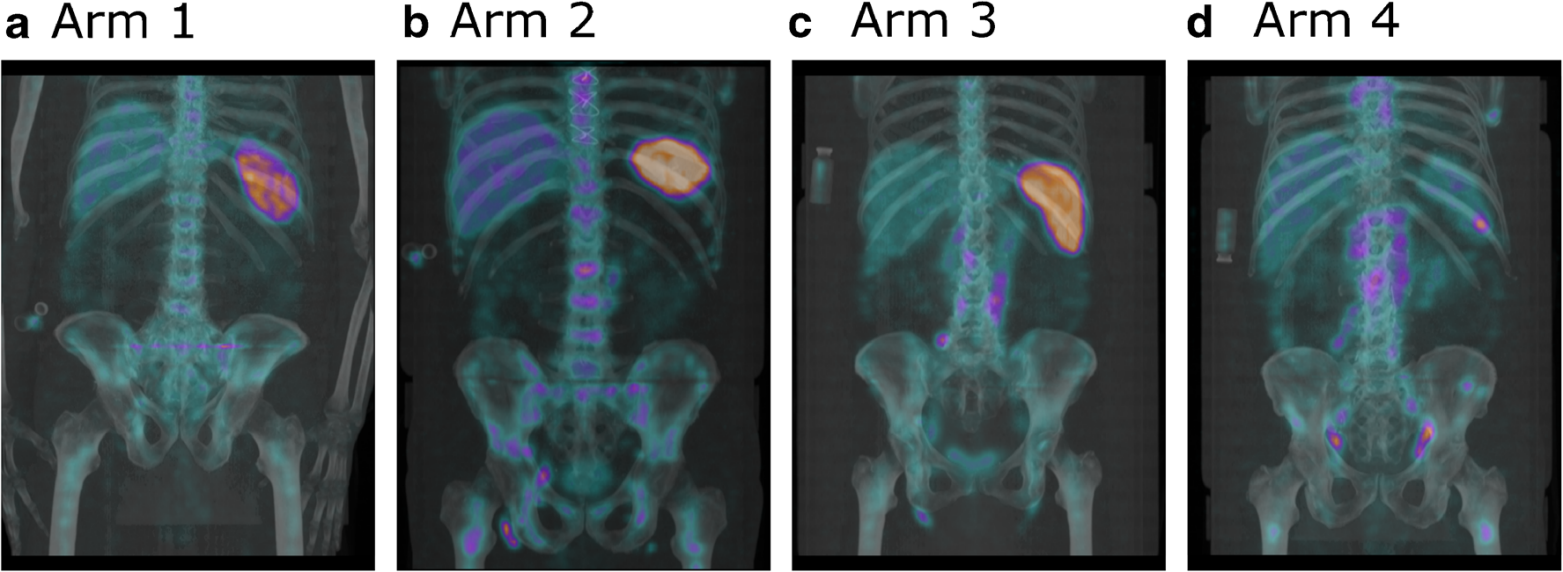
Fused SPECT/CT maximum intensity projection images at 96 h after injection of ^177^Lu-lilotomab satetraxetan. a–d Patients 009 (arm 1; 40 mg lilotomab), 013 (arm 2; no lilotomab), 017 (arm 3; no lilotomab), and 019 (arm 4; 100 mg lilotomab per m^2^ BSA) are shown. After 96 h, most of the ARC has been washed out of the blood, and ^177^Lu-lilotomab satetraxetan uptake is observed in tumors, liver, spleen, and bone marrow. A shift in uptake for the spleen can be observed when an increased amount of pre-dosing with lilotomab is given. Abdominal and inguinal lesions are visible, and the arm 4 patient also had a focal lesion in the spleen. All four patients had received 15 MBq/kg body weight of ^177^Lu-lilotomab satetraxetan, and the same intensity scale is used for all images
